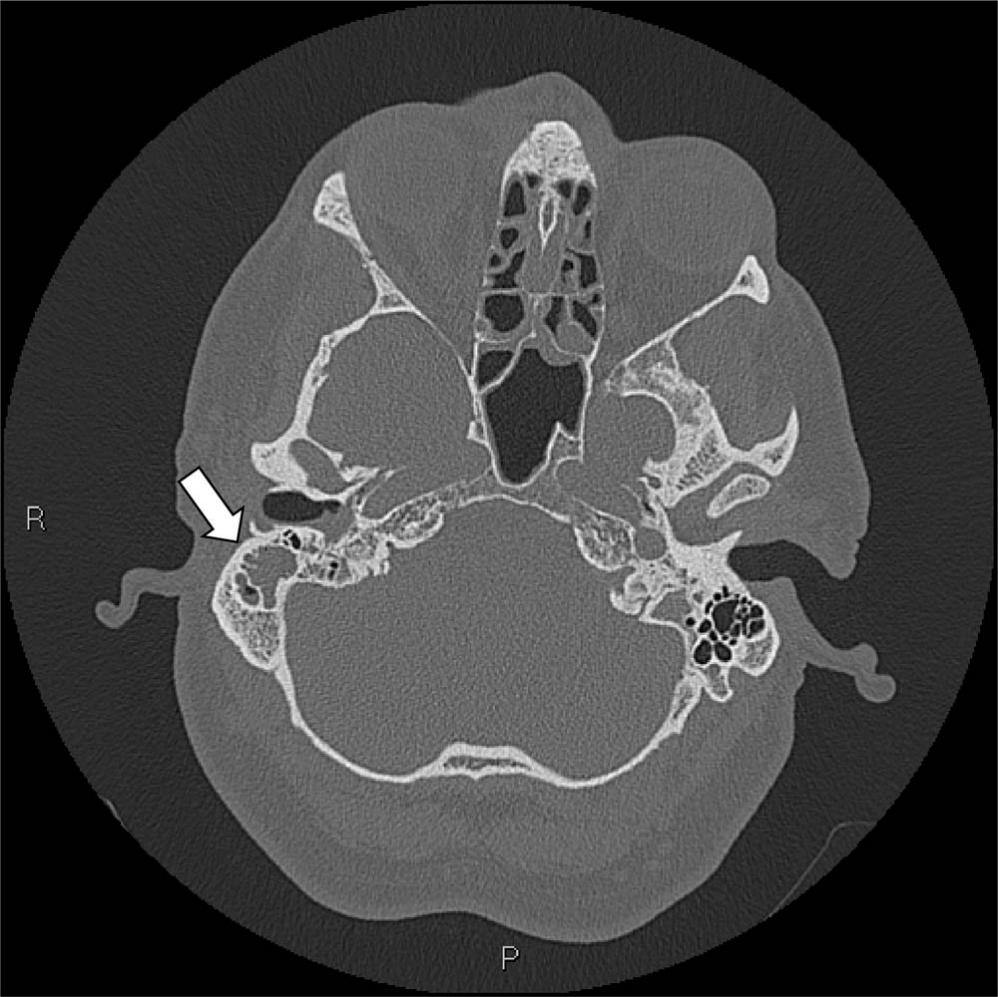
Axial slice CT brain (bone window): Loss of aeration and necrosis within the right-sided mastoid air cells (white arrow), suggesting the presence of fluid-filled cavities from an infective and inflammatory process.
